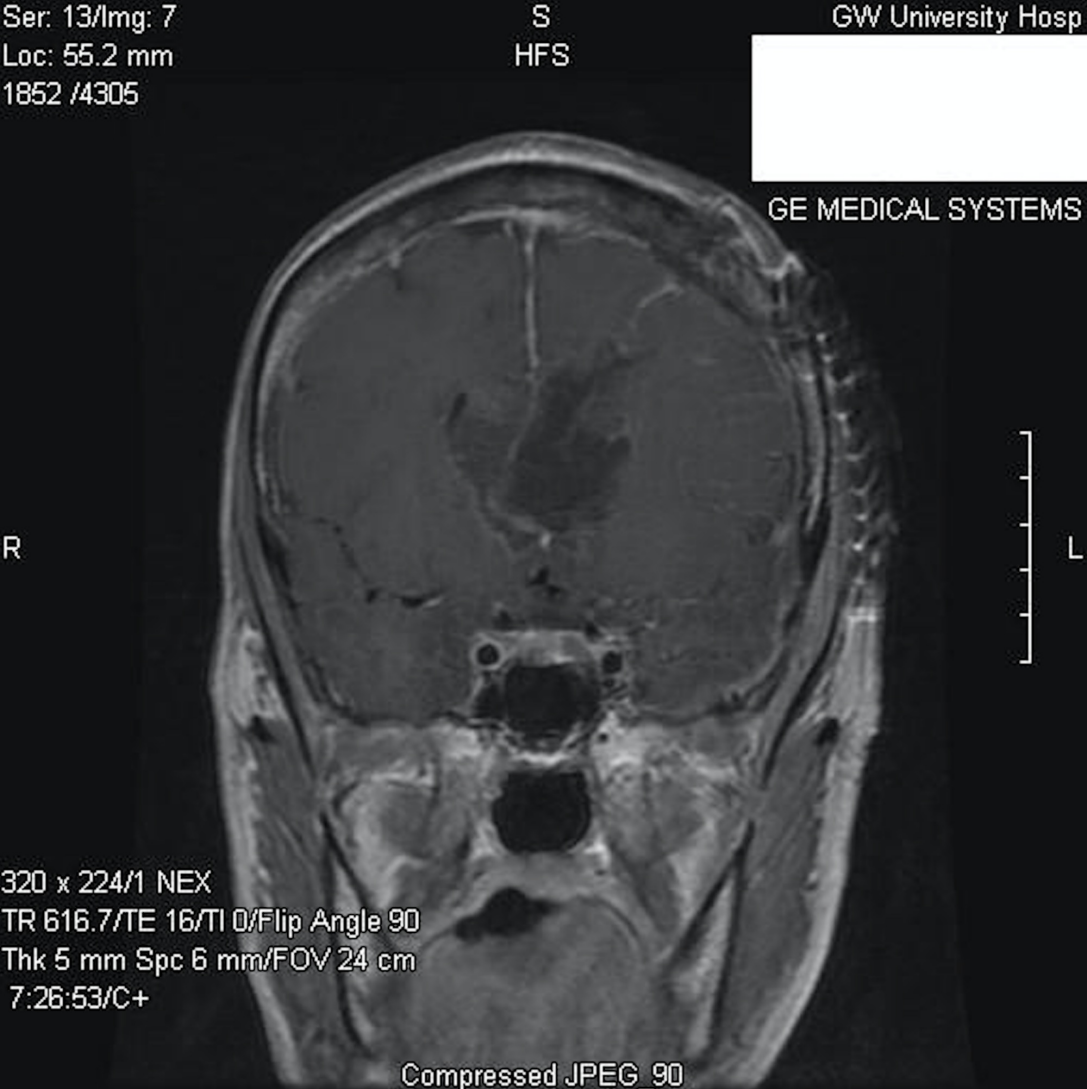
Postoperative coronal post-contrast T1-weighted MR image MR: magnetic resonance
